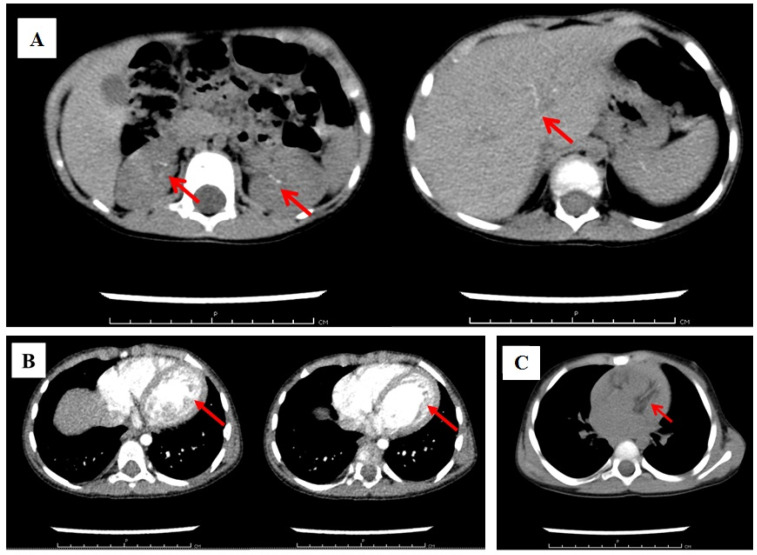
Results of multispiral computed tomography with intravenous contrast. Fragmentary calcification of hepatic, renal, and splenic arteries. The red arrow is pointing at the calcification (A); the red arrow is pointing at noncompact myocardium of the left ventricle and its dilation (B); the red arrow is pointing at calcification of the coronary arteries (C).
